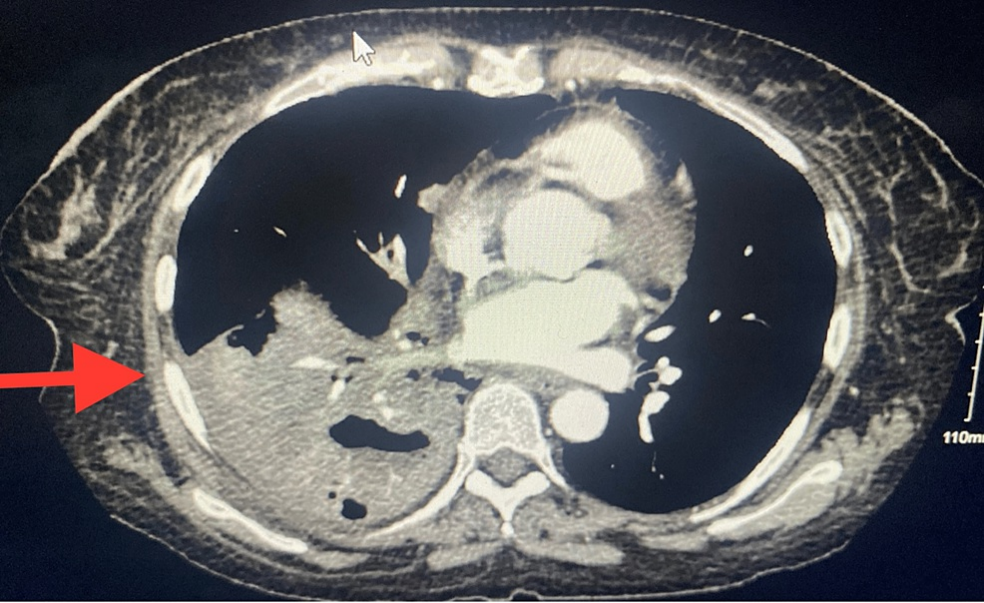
CT chest showing right lower lobe consolidation with cavitation suspect necrotizing pneumonia.
